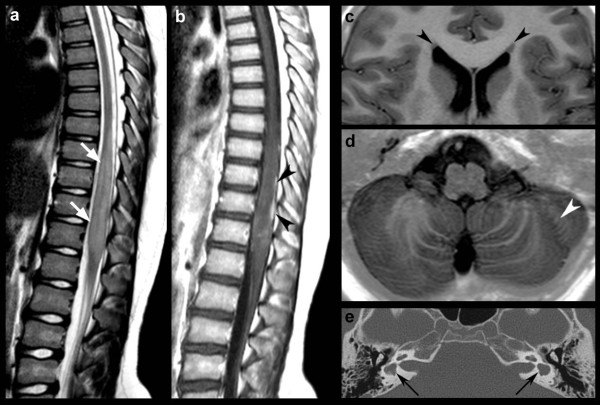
Craniospinal MRI (a-d) and temporal bone CT (e) images. Spine MRI reveals a solid tumour mass hyper intense on sagittal T2-weighted images (a, white arrows) with mild and inhomogeneous contrast enhancement on Gd-enhanced sagittal T1-weighted images (b, black arrowheads). Brain MRI axial STIR show bilateral frontal periventricular nodules of gray matter heterotopias (c, black arrowheads) and an area of abnormal cerebella foliation (d, white arrowhead). Temporal bone CT scan depicts bilateral digenesis of the lateral semicircular canals and vestibules forming a single cavity with normal appearing cochleae (e, black arrows).
